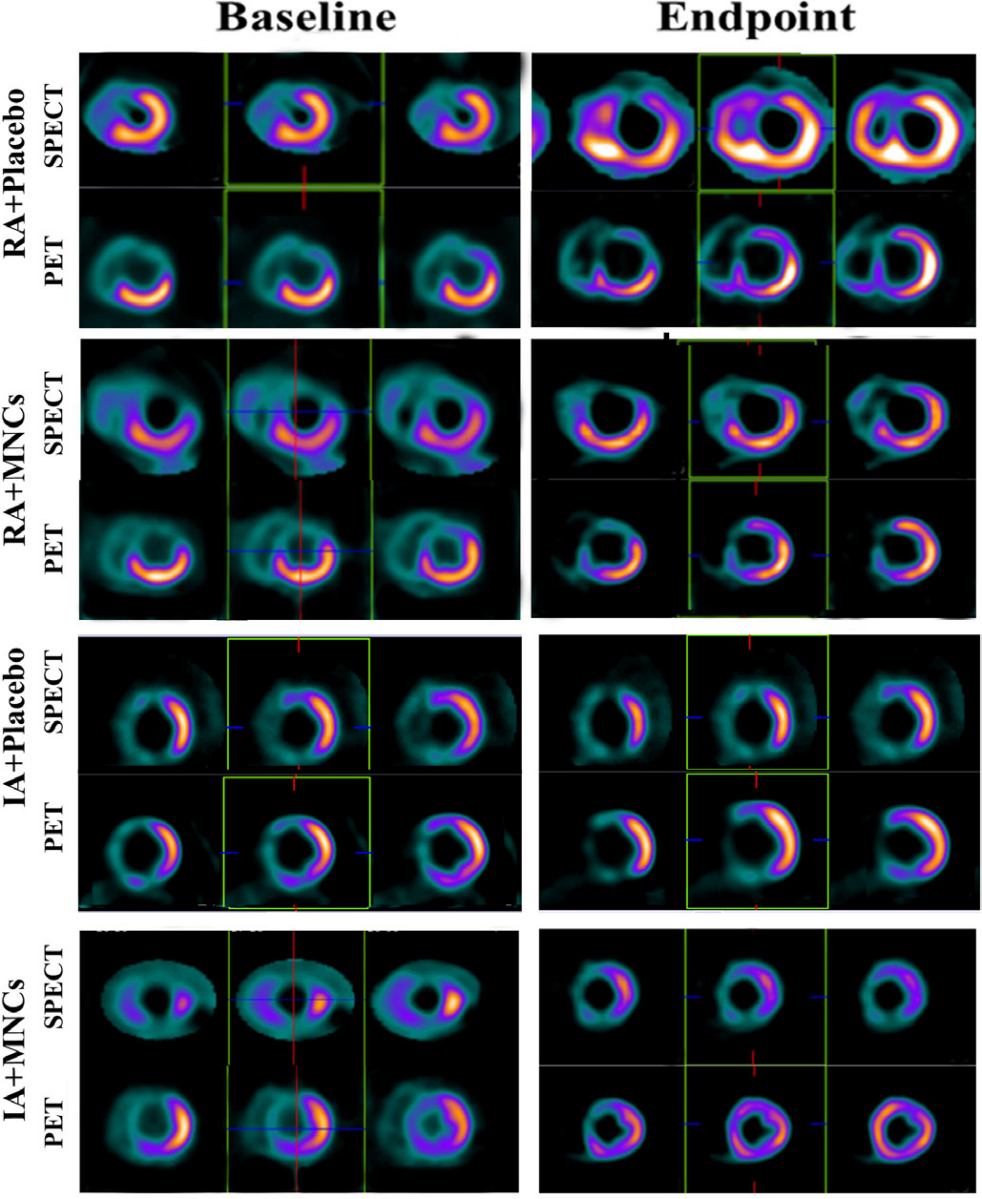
The representative images of SPECT and PET denoting myocardial regeneration after combined treatment with intensive atorvastatin (IA) and MNCs in the same patient shown in figure 2. A, intensive atorvastatin; MNCs, mononuclear cells; PET, positron emission tomography; RA, regular atorvastatin; SPECT, single-photon emission CT
